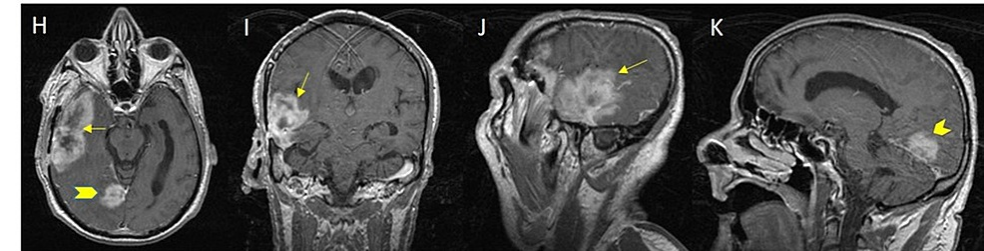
Axial (H), coronal (I) and sagittal (J, K) T1 images from post contrast MRI demonstrate an aggressive appearing markedly heterogenous large mass in the right middle cranial fossa involving the right temporal lobe (arrow). An additional separate lesion is seen adherent to the right side of the tentorium (arrowhead). This was found to be a recurrent glioblastoma multiforme mimicking as hemorrhage before.
